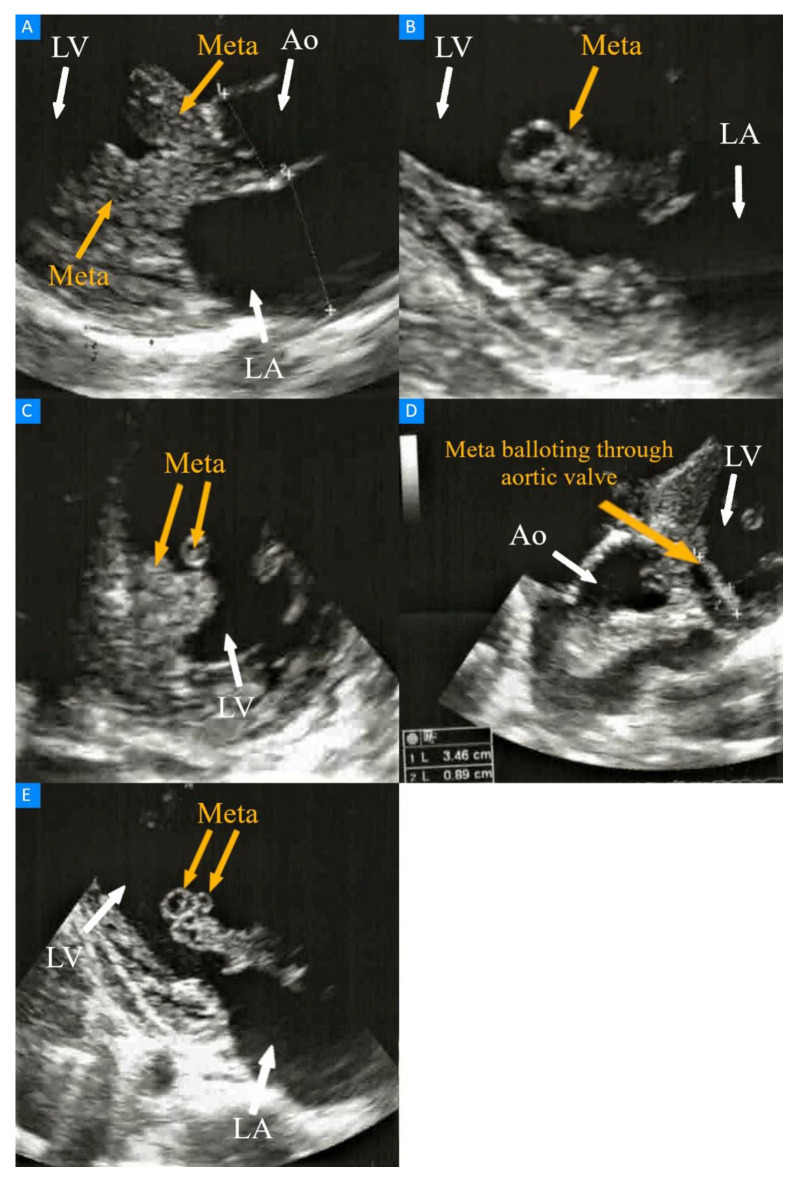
Transthoracic echocardiography visualizing metastases of ccRCC to the heart. (A) Modified parasternal long-axis view, metastasis in the left ventricle infiltrating interventricular septum; 1—the dimension of the aortic root, 2—the dimension of the left atrium. (B) Modified parasternal long axis view, metastasis in the left ventricle. (C) Parasternal short axis view, metastasis infiltrating the mitral valve. (D) Modified apical 5 chamber view visualizing the mobile metastatic lesion of 35 mm × 8 mm which balloted through the aortic valve. (E) Modified apical 3 chamber view visualizing two round and mobile metastases.
